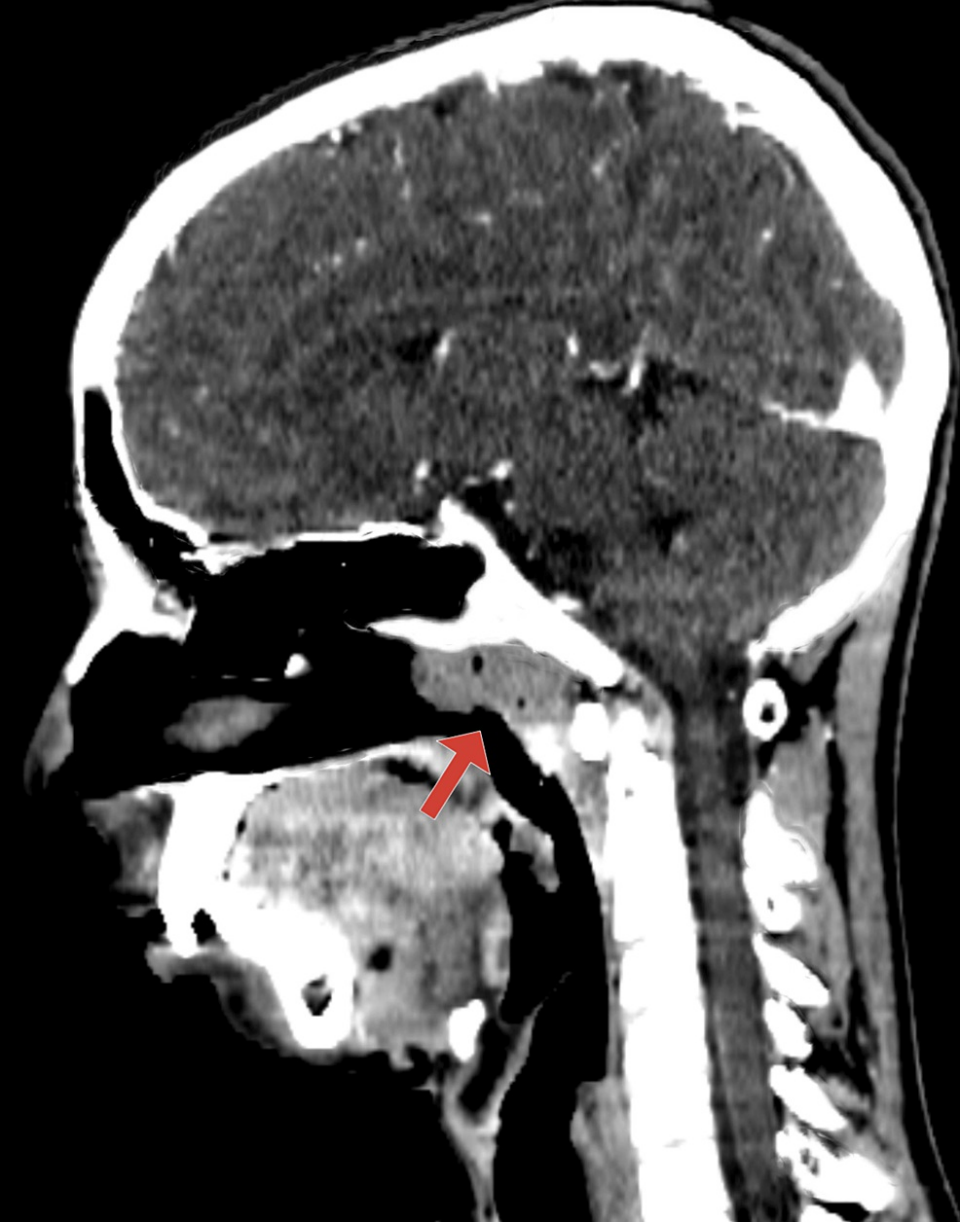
CT demonstrates a heterogeneously enhancing mass (red arrow) occupying the nasopharynx
